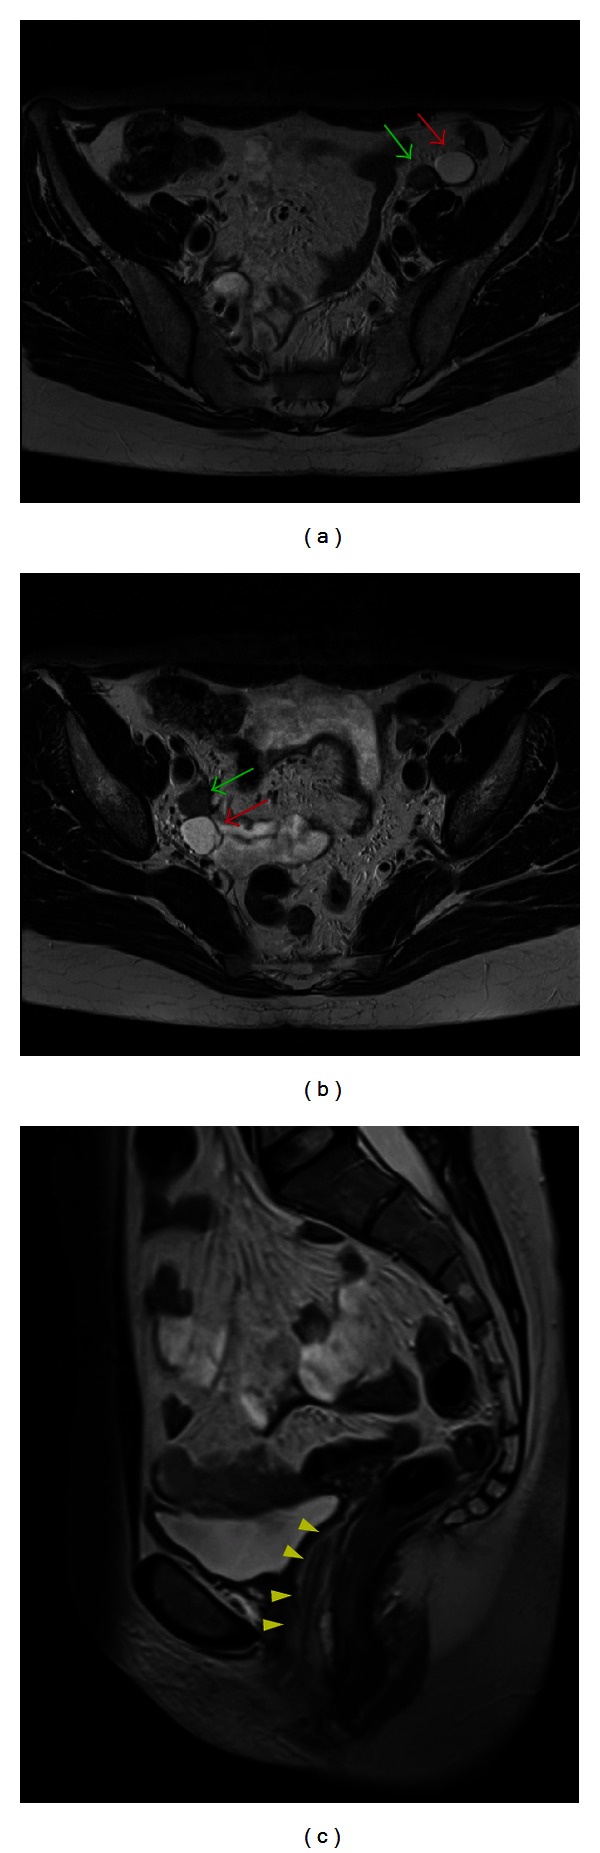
A 34-year-old female with CAIS syndrome. The axial T2-weighted images ((a) and (b)) show a left testis along the left external iliac artery and a right testis in the right obturator canal (green arrows) with adjoining cysts (red arrows). Sagittal image (c) shows absence of Müllerian structures and presence of the lower vagina (yellow arrowheads) (3T magnetic resonance imaging. Axial 4 mm fast T2-weighted images, TR/TE 5030/119 ms; sagittal 5 mm HASTE-sequence, TR/TE 6500/89).
